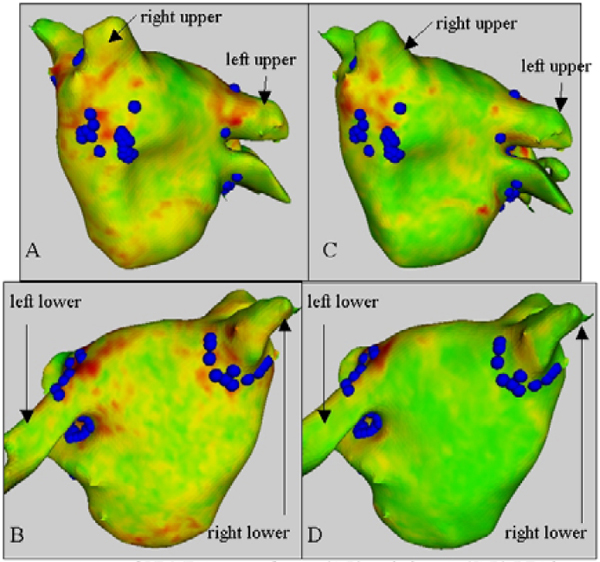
3D representation of LE MR images of acute (A-B) and chronic (C-D) RFA lesions. Overlaid in blue are the ablation points acquired with NavX. It can be seen in the chronic image that an area of enhancement is no longer apparent around the right lower PV.
